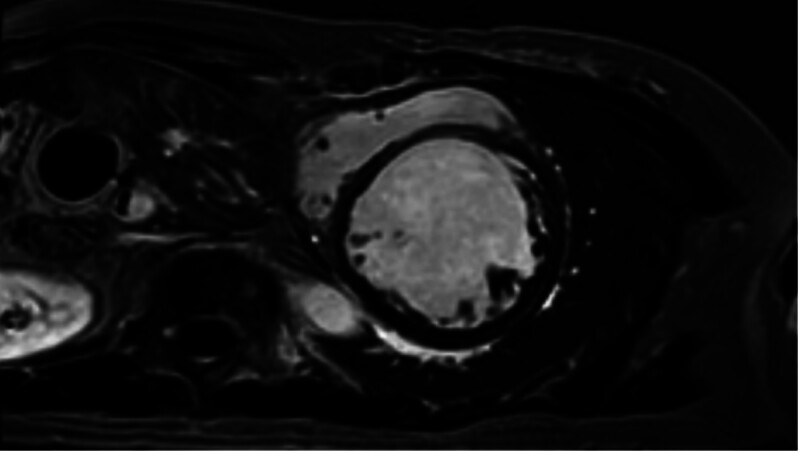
Cardiac MRI. Cardiac MRI performed on August 30, 2024, showing global cardiac enlargement, diffuse thinning of the left ventricular wall, and linear mid-myocardial fibrosis in the interventricular septum. The LVEF was estimated at 24%. LVEF = left ventricular ejection fraction, MRI = magnetic resonance imaging.
